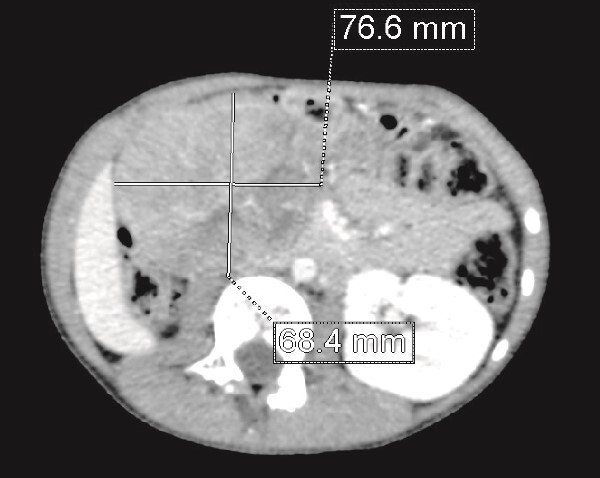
CT scan: A solid mass of 9 × 6 cm of diameter containing some areas of necrosis, well circumscribed, well encapsulated at the expense of the right kidney in contact with the cephalic portion of the pancreas and in close contact with the inferior vena cava, which is pushed to the left. The right kidney is not visualized.
